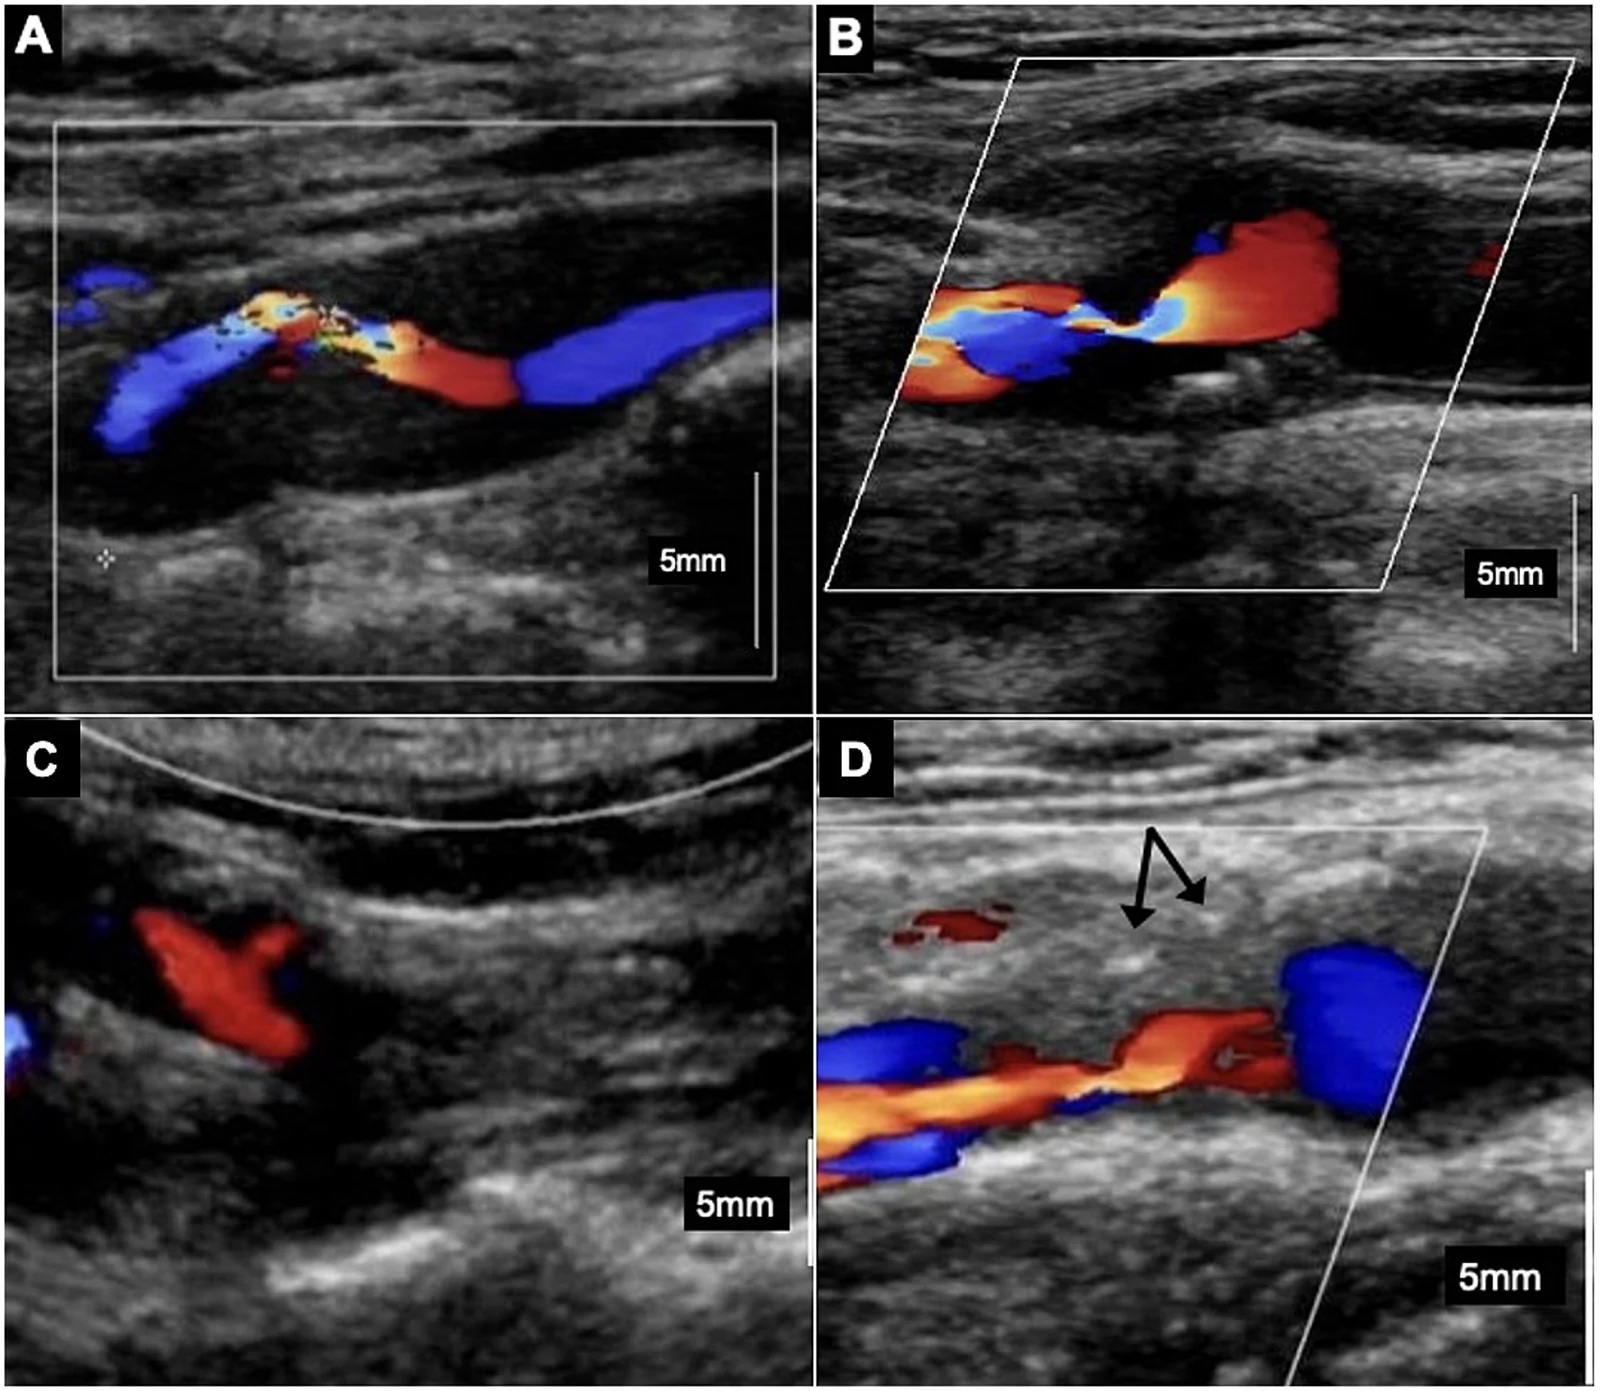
Carotid plaque juxtaluminal echolucency, Discrete White Areas and ulceration. A, B: Two patients with carotid plaques displaying juxtaluminal echolucency – i.e. echolucent plaque adjacent to the lumen on ultrasound. C: Patient with carotid stenosis by a plaque complicated with ulceration from the lumen into the plaque with doppler flow on ultrasound. D: Patient with a carotid plaque that contains two hyperechogenic Discrete White Areas (DWA) without acoustic shadowing on ultrasound.
